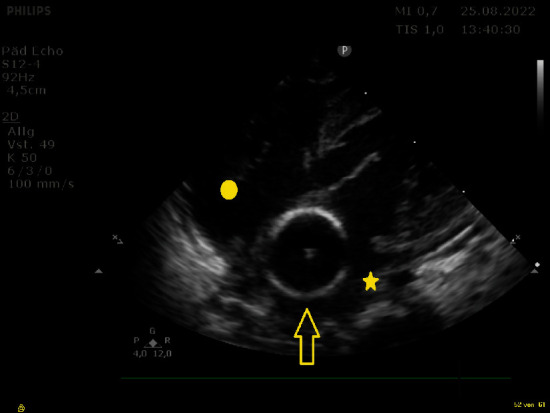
Echocardiography of the same patient with the balloon inflated (arrow) in the left atrium (star). Dot: right atrium.
